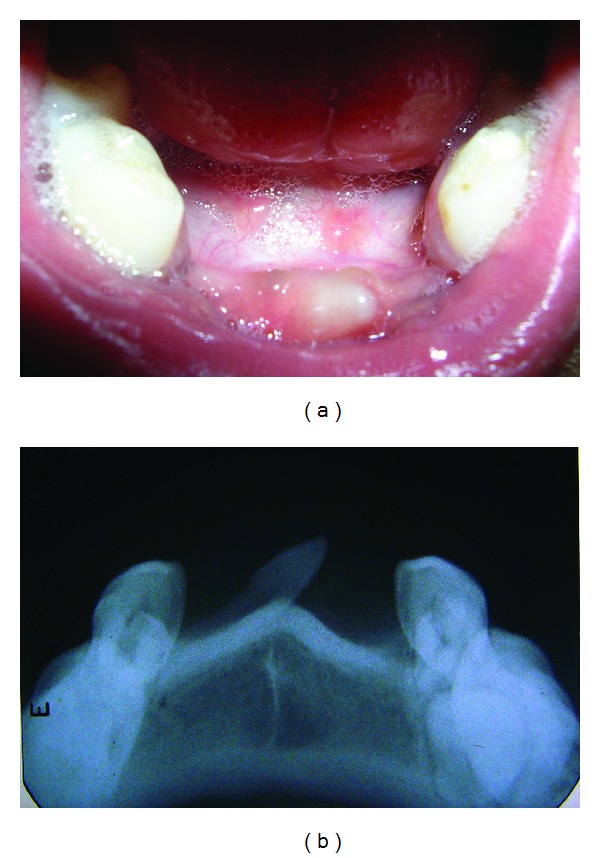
(a) Showing mesiodens in lower arch. (b) Radiograph showing mesiodens in lower arch.
